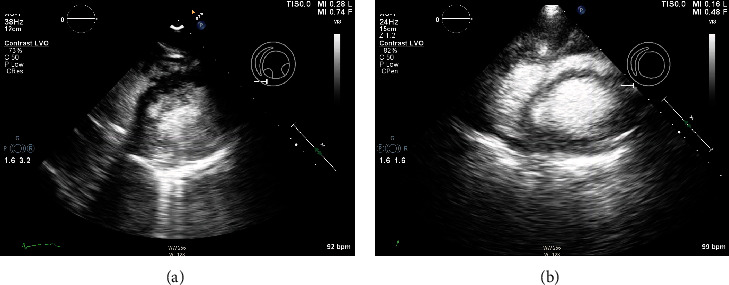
(a–b) Short axis view with ultrasound-enhancing agent demonstrating profound biventricular hypokinesis. (b) Short axis view with ultrasound-enhancing agent demonstrating recovery.
